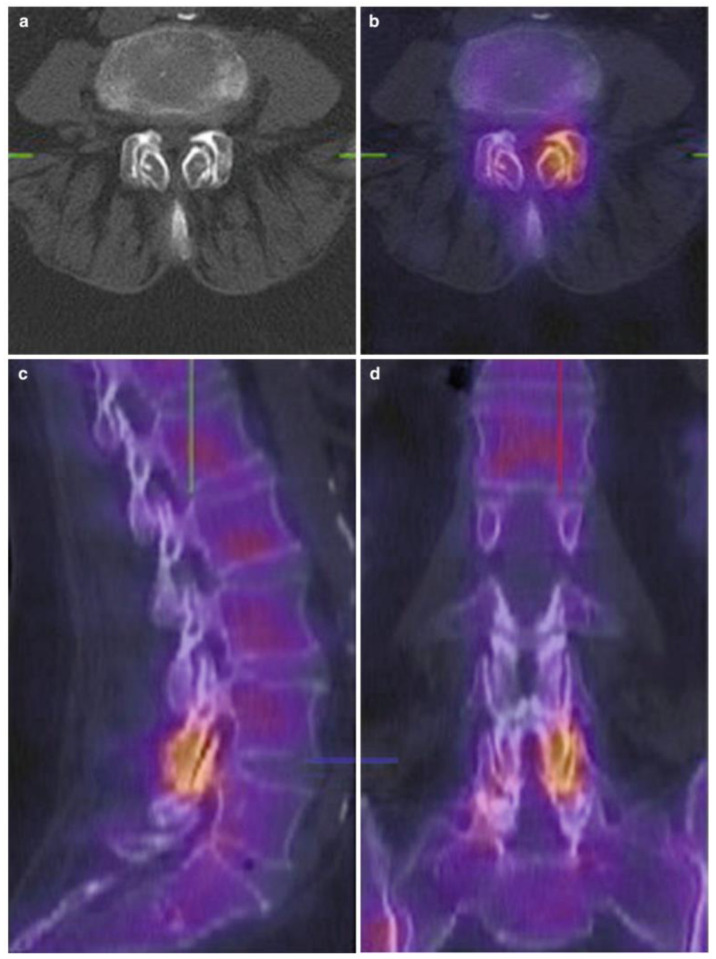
SPECT-CT of the lumbar spine. Woman with low back pain and a typical presentation of facet joint osteoarthritis on SPECT/CT at the L4–L5 level. Axial CT image (a) and axial (b), sagittal (c), and coronal (d) SPECT/CT images. Courtesy by E.V.d.Kelft.
